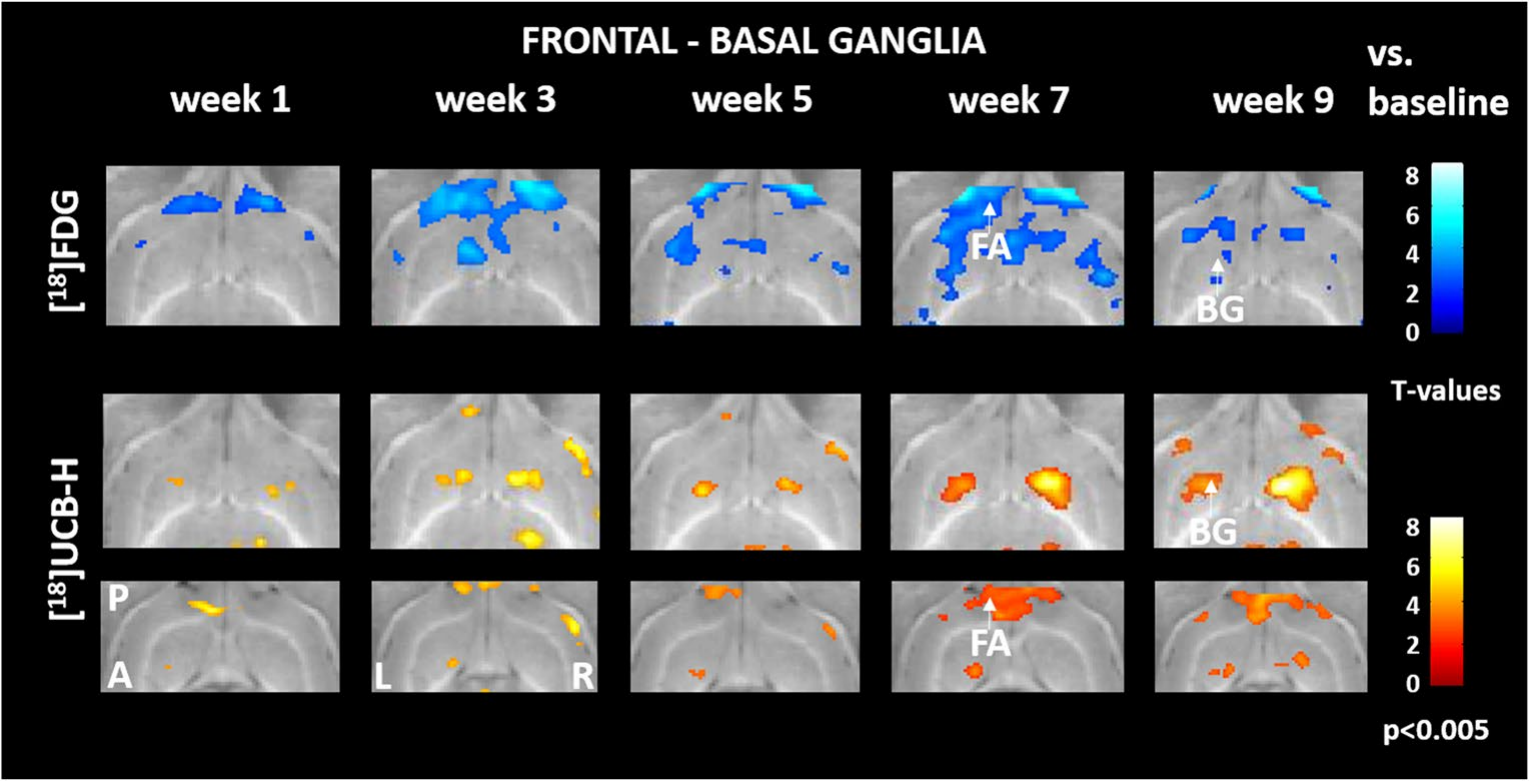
Comparison of regional cerebral glucose metabolism and synaptic density in frontal–basal ganglia networks. Reduced [^18^F]FDG uptake in the frontal association cortex and striatum was paralleled by an increase in [^18^F]UCB-H binding. A: anterior, P: posterior, Le: left, Ri: right, BG: basal ganglia, FA: frontal association cortex, [^18^F]FDG: [^18^F]fluorodeoxyglucose. Changes against baseline are depicted as t-values at a significance level of *p* < 0.005.
